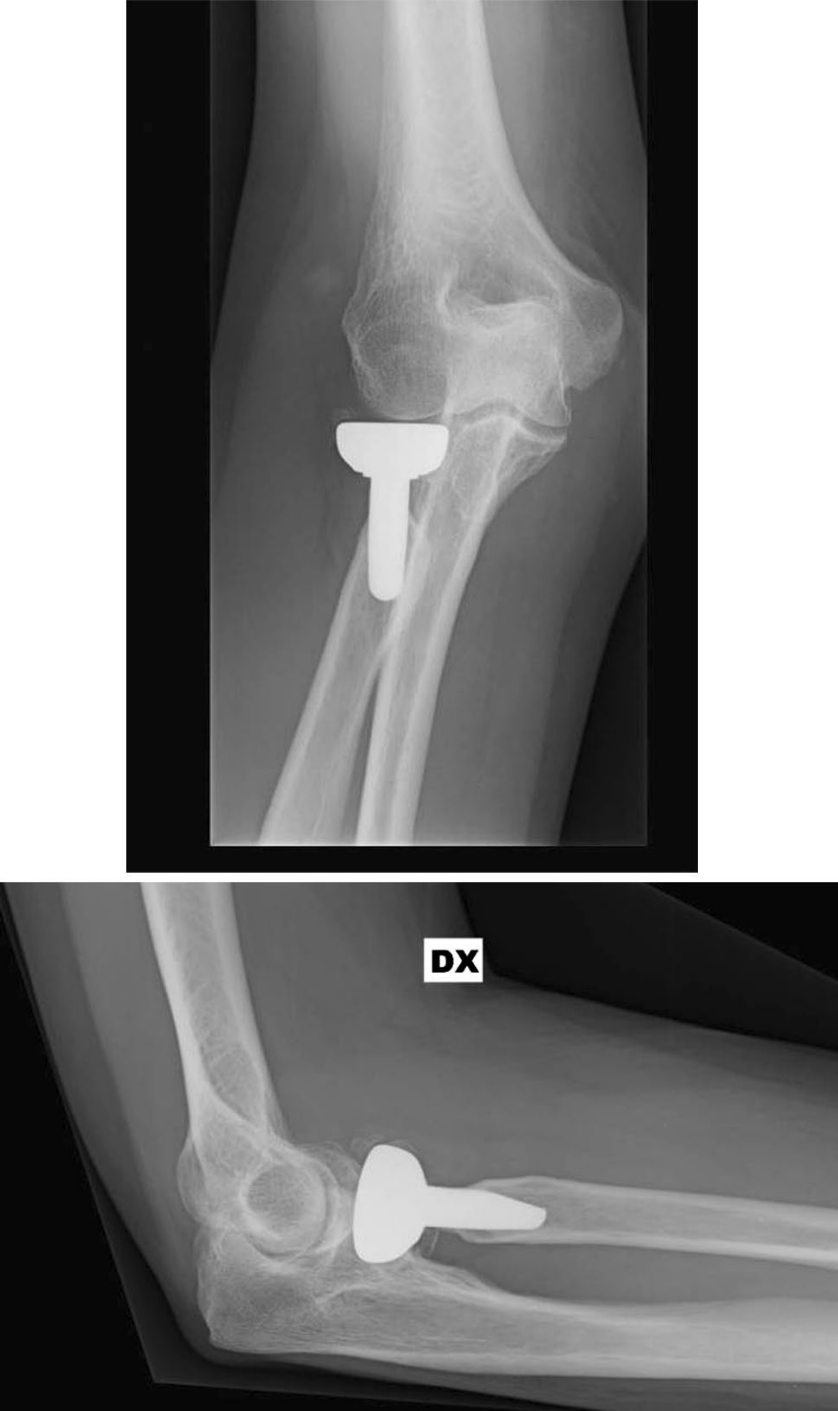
48 months X-ray follow-up of the same patient showing stress shielding radial neck resorption
